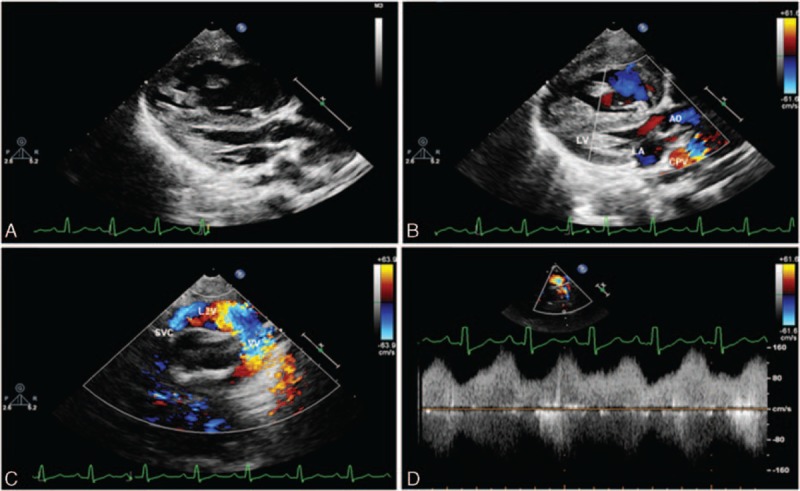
Transthoracic echocardiography shows supracardiac total anomalous pulmonary venous connection drainage to superior vena cava (SVC) via left innominate vein (LIV) of a 6-month-old boy. (A) Two-dimensional view of the parasternal long axis shows a small left side of heart, hypertrophy of right ventricle, and the common pulmonary vein (CPV) behind the left atrium (LA). (B) Color Doppler shows the flow in the CPV and no flow spray from CPV to LA. (C) Suprasternal view—the flow from CPV to SVC via vertical vein (VV) and LIV shows clearly by color Doppler. (D) Pulse Doppler shows the phasic flow pattern in VV, varying with the cardiac cycle.
